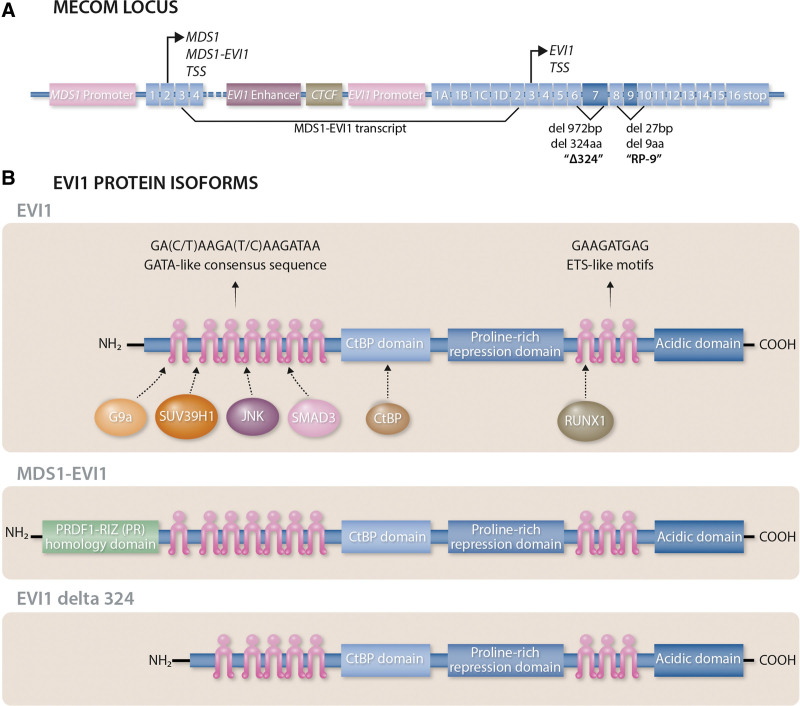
The MECOM locus and EVI1 protein isoforms. (A) The MECOM locus: The *MDS1 and EVI1 complex locus* (*MECOM*) encodes several transcripts and protein isoforms through differential splicing and the presence of 2 distinct transcription start sites (TSS), each with their own promoter region, located either upstream of the *MDS1* cDNA or upstream of the *EVI1* cDNA. The *MDS1* TSS generates the MDS1 protein and the MDS1-EVI1 protein via alternative splicing of exon 3 of *MDS1* to exon 2 of *EVI1*. Alternative splicing events of *EVI1* include a deletion of 972bp within exon 7, resulting in the so-called *EVI1* delta 324 isoform, as well as an exon skipping event of the 27bp long exon 9, resulting in the so-called RP-9 isoform. (B) EVI1 protein isoforms: The EVI1 protein has 2 distinct zinc finger domains. ZF1, containing 7 zinc finger repeats, is located at the N-terminus of the protein and binds to GATA-like consensus sequences. The more distal ZF2, containing 3 additional zinc finger repeats, binds to ETS-like motifs. EVI1 can interact with the histone methyltransferases G9a and SUV39H1 via ZF1, as well as the protein kinase JNK and the transcription factor SMAD3. EVI1 additionally possesses domains to directly interact with the transcriptional corepressor CtBP and the transcription factor RUNX1. Alternative splicing of exon 3 of *MDS1* to exon 2 of *EVI1* results in the N-terminal addition of the PRDF1-RIZ (PR) homology domain in the MDS1-EVI1 protein. The deletion of 972 bp within exon 7 of *EVI1* leads to a deletion of 324 amino acids and the so-called EVI1 delta 324 protein isoform lacking zinc finger motifs 6 and 7 within ZF1.
